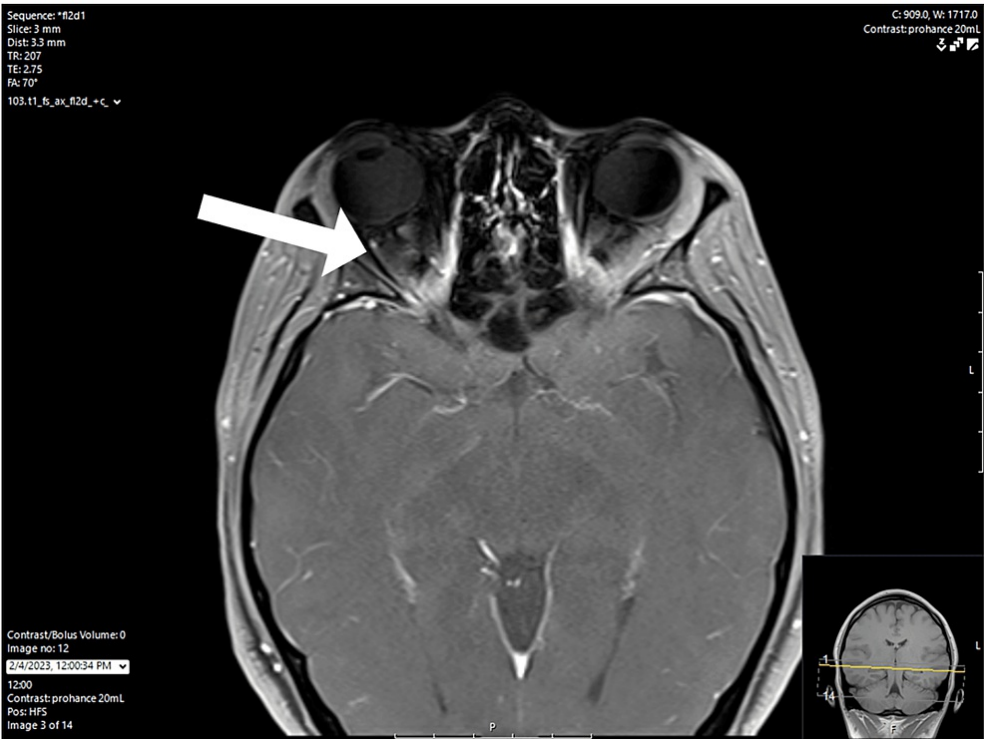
MRI of the face, neck, and orbit with/without contrast MRI: magnetic resonance imaging
